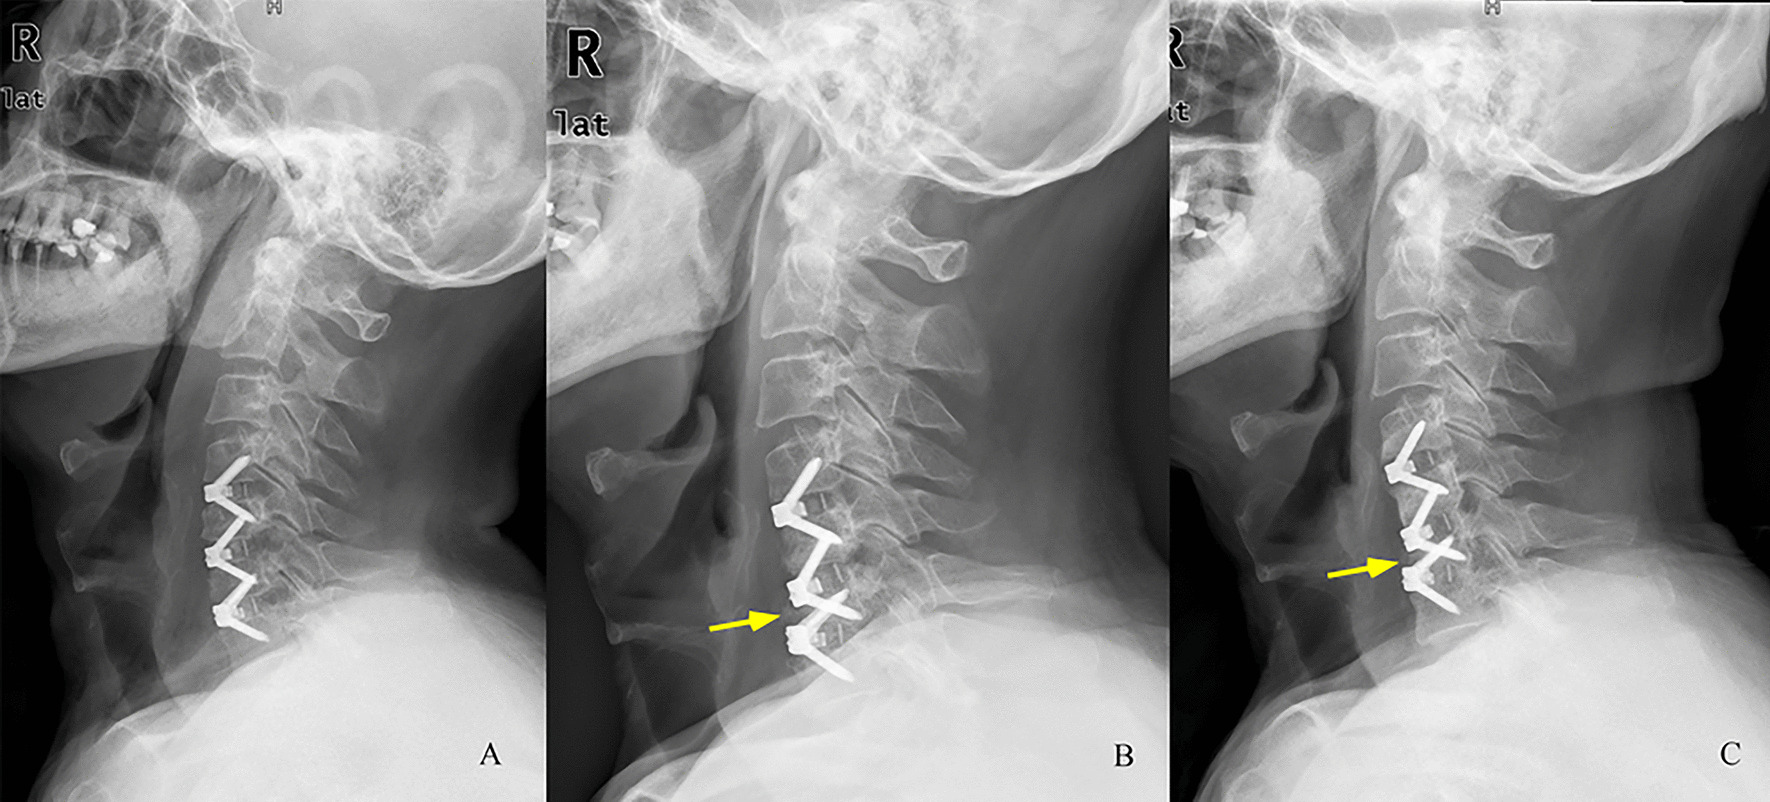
A 68-year-old man underwent three-level anterior cervical discectomy and fusion (ACDF) with Zero-p implant system. The lateral X-ray 3 days after surgery showed the prostheses were implanted well (A). However, the severe collapsibility phenomenon could be observed at C6 vertebral body 3 months postoperatively (B). The lateral X-ray 18 months postoperatively showed the collapsibility didn’t become aggravated after the solid fusion achieved (C)
